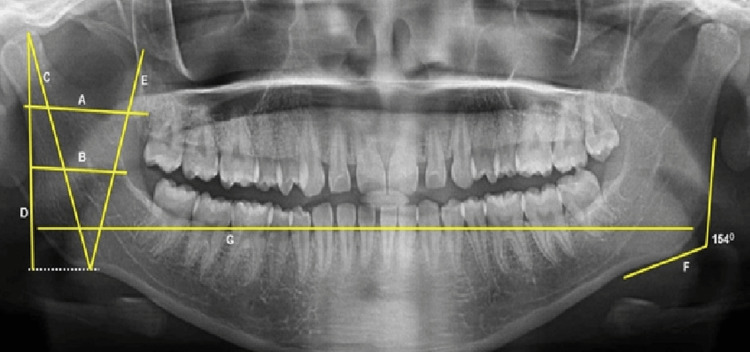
Image showing OPG measurements. OPG, orthopantomograph
